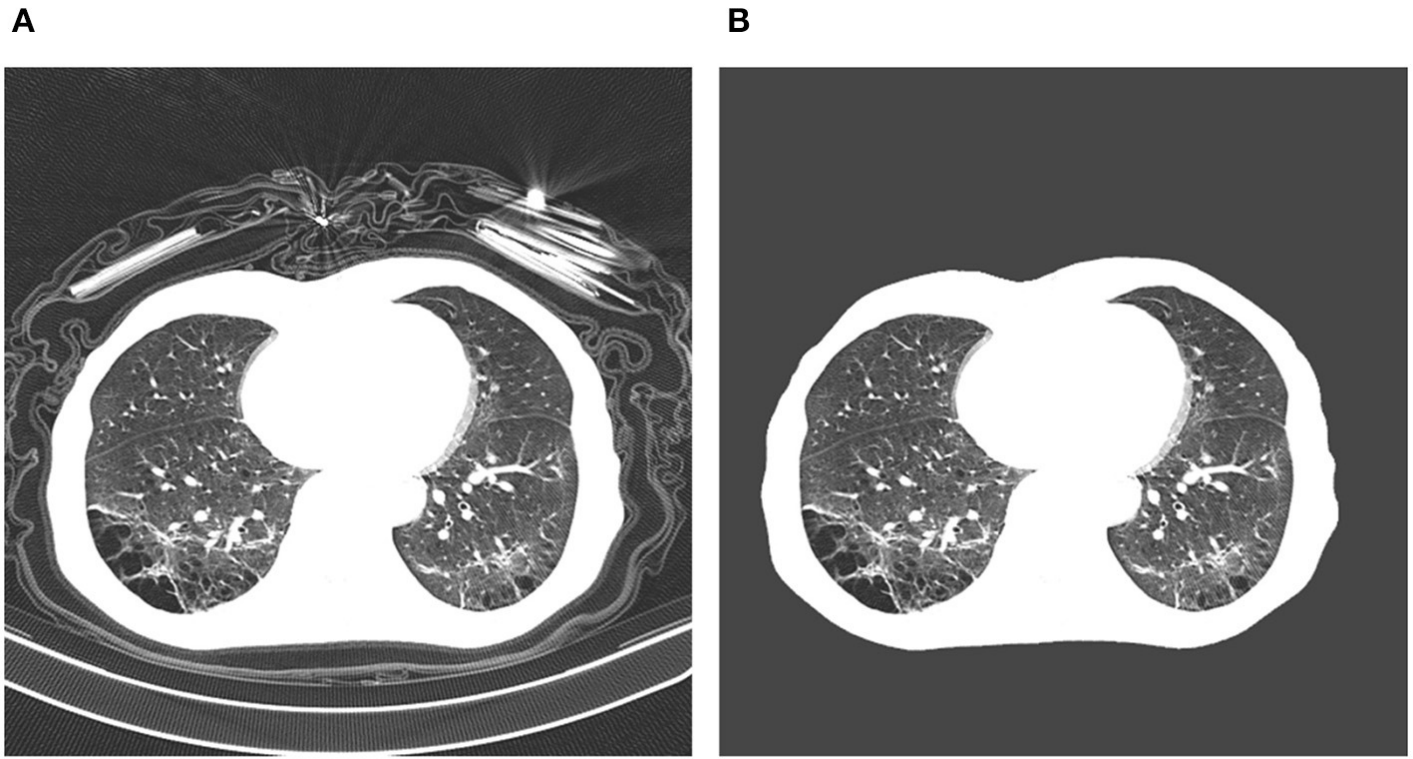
Example COVID-19 case before and after removal of irrelevant visual indicators as part of data augmentation. In (A), a number of irrelevant visual indicators are present, such as the patient table of the CT scanner as well as imaging artifacts. After removing these irrelevant indicators, the image in (B) is obtained.
